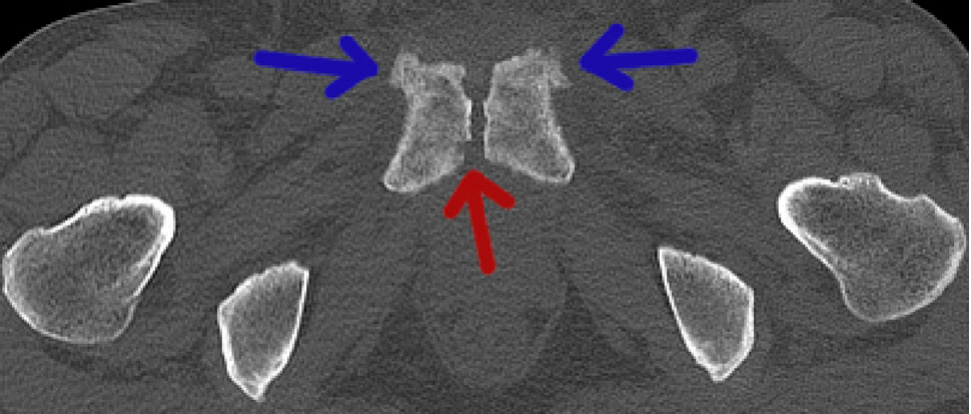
Computed tomography showing degenerative changes accompanied by erosion of the pubic symphysis (red arrow) and pubic osteophytes (blue arrows).
